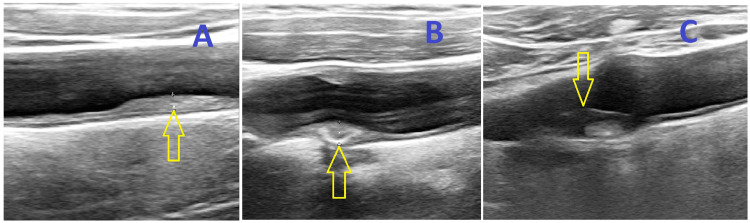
Duplex ultrasonography (B-mode image) shows different types of carotid plaques (arrows). Homogenous (A), calcified (B), and mixed (C).
